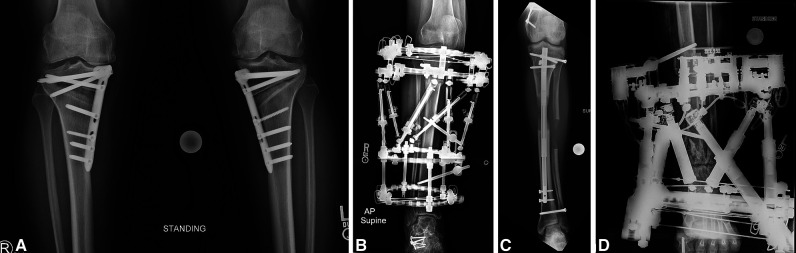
Radiographs showing the examples of osteotomies and fixation included in this study. A, A 52-year-old woman with staged bilateral high tibia osteotomies, fixated with plate and screws, to address tibia-based genu varum; she did not have any fasciotomy. B, A 65-year-old woman with right proximal metaphyseal tibia osteotomy and mid-diaphyseal fibula osteotomy, fixated with a dynamic hexapod external fixator, to address torsion; she had an anterior compartment fasciotomy. C, A 21-year-old man with left diaphyseal tibia and fibula osteotomy, fixated with dynamic nail, to address short stature related to hypophosphatemic rickets; he had anterior and lateral compartment fasciotomies. D, A 41-year-old woman with left supramalleolar tibia and fibula osteotomy, fixated with a dynamic hexapod external fixator, to address a multiplanar deformity; she did not have any fasciotomy. The radiographically dense struts and wires are part of a robotically self-controlled programmable adjustment system.
